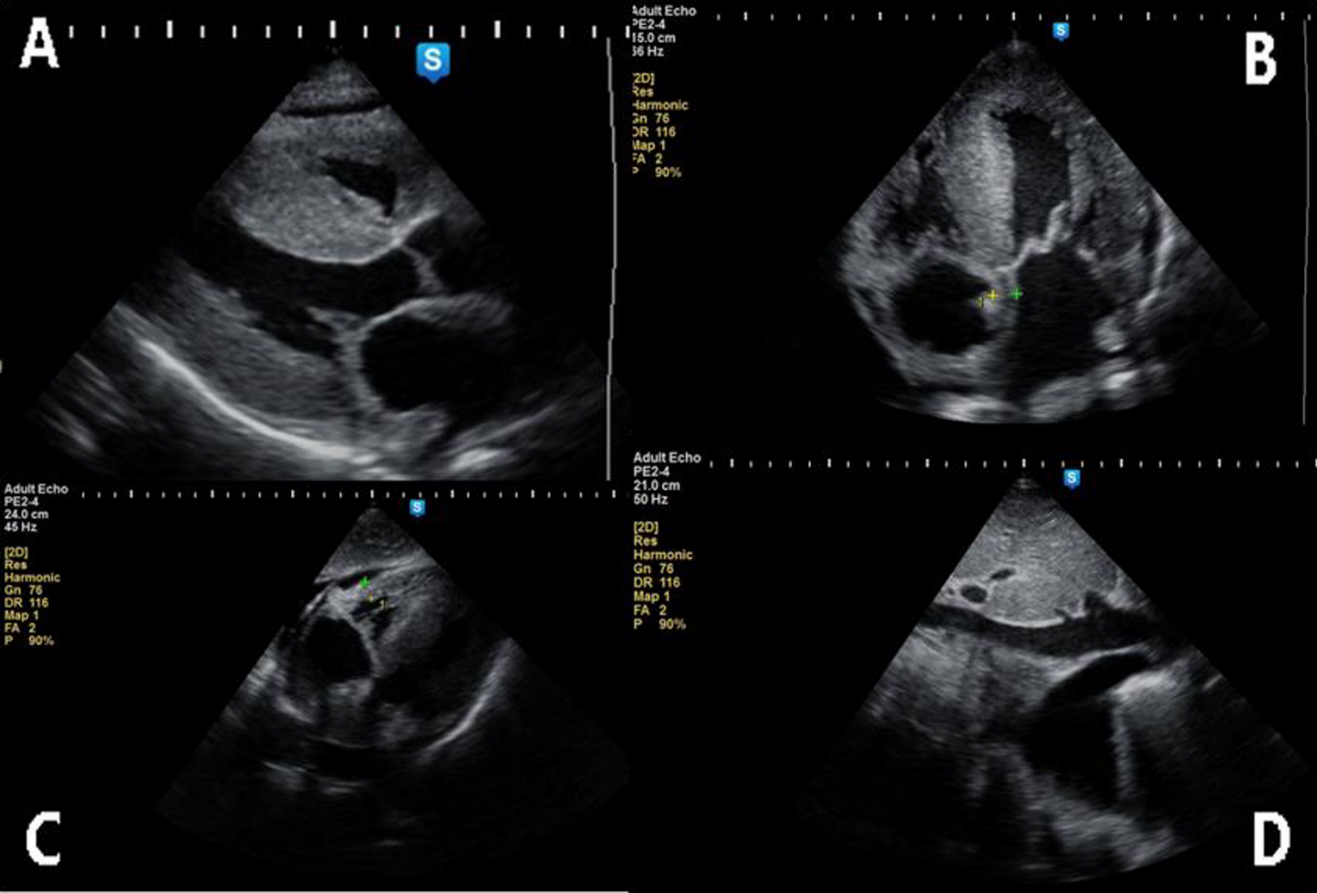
Transthoracic echocardiography (TTE): parasternal long axis showing severe left ventricular hypertrophy with small anterior pericardial effusion (A), marked septal hypertrophy, biatrial enlargement and interatrial septal hypertrophy with the granular sparkling of the myocardium on apical four‐chamber view (B), right ventricular hypertrophy with small pericardial effusion in subcostal view (C), dilated inferior vena cava (IVC) with right side pleural effusion (D).
